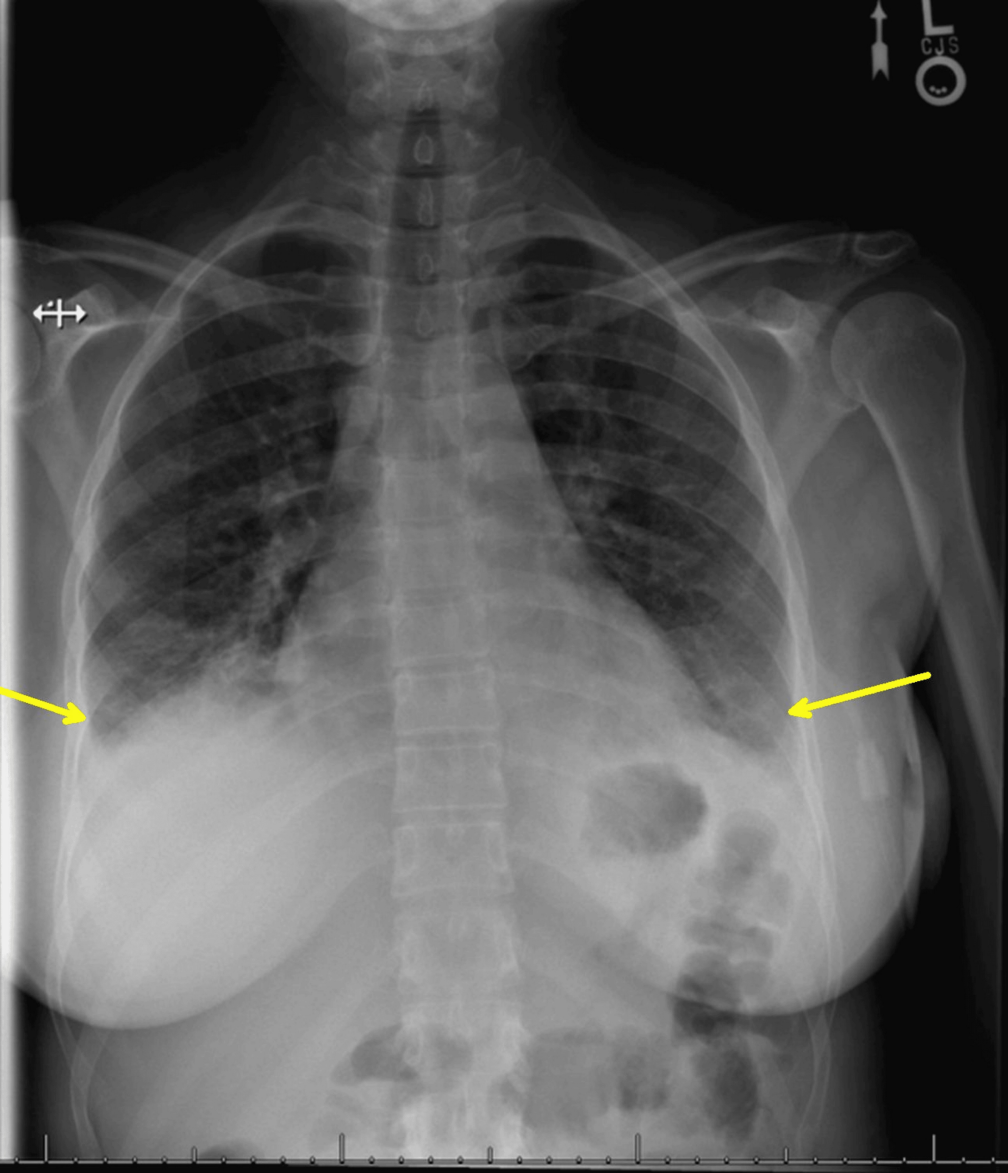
Chest radiograph demonstrating dependent pleural effusions (yellow arrows) and patchy bilateral alveolar pulmonary opacities.
